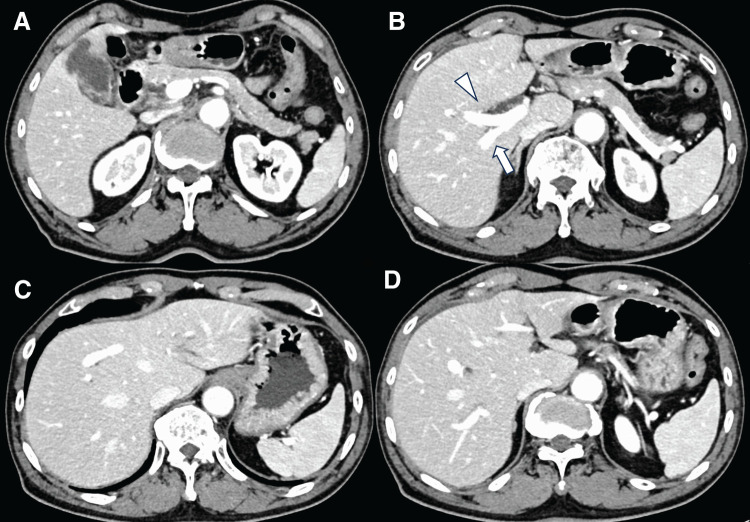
CECT revealed gallbladder carcinoma and the absence of a PV. (A) The CECT scan indicates the gallbladder that infiltrated the gallbladder bed and liver parenchyma. (B–D) The CECT scan shows the intrahepatic path of the main trunk of the PV. The portal ring runs through the right liver and inflows the left liver. The white arrowhead indicates the anterior PV and the white arrow indicates the posterior PV. CECT, contrast-enhanced CT; PV, portal vein
